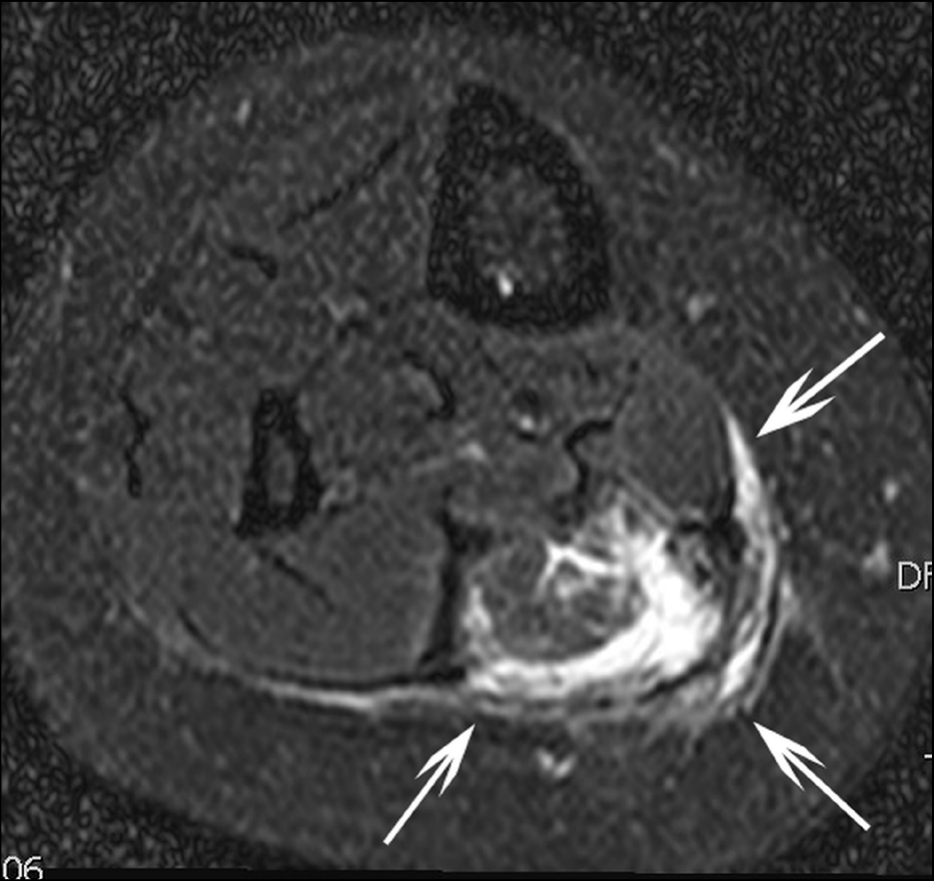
T2-weigthed axial magnetic resonance image in the same patient three months after percutaneous radiofrequency ablation which was performed after two failed percutaneous sclerotherapies with sotradecol. Hyperintense rim (white arrows) around the ablated area suggestive for perileasional oedema.
